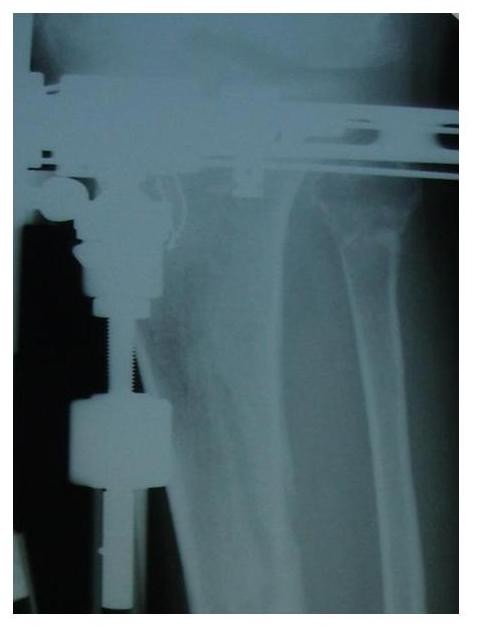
Postoperative lateral x-ray of the same patient.
